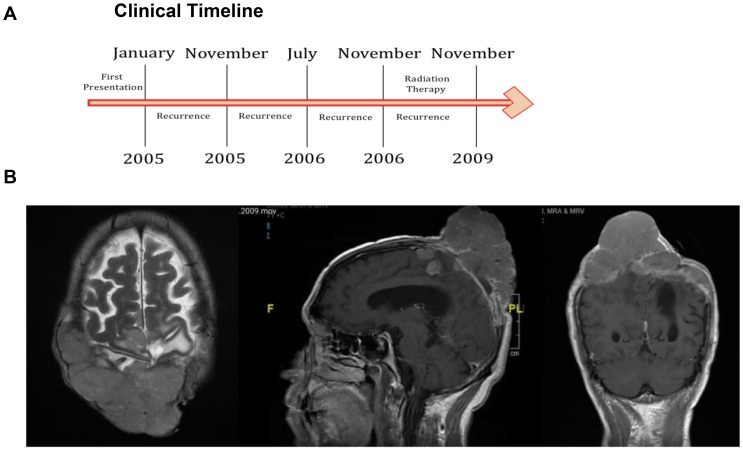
TERTp mutation identified in a single case. (A) Clinical timeline of the identified patient. (B) Pre-operative MRI of axial, sagittal and coronal views of the patient.
